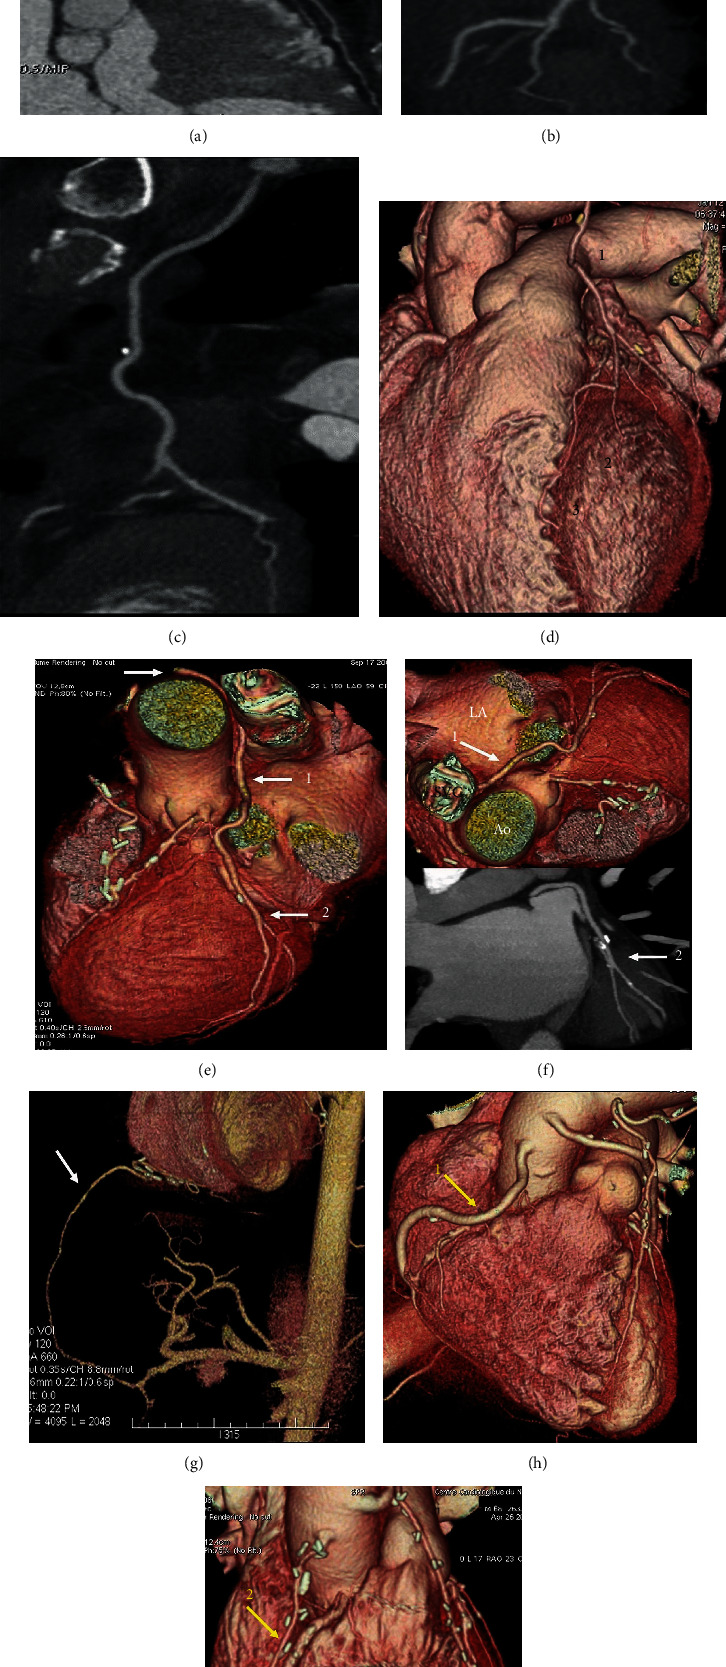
(a–i) Postprocessing of CT angiography of CABG by mean of volume rendering and 2D curved imaging with automatic tracking. (a–c) show 2D curved imaging of CABG with automatic tracking. (a) LITA anastomosed on LAD. (b) LITA sequential grafting on LAD and first diagonal branch. (c) RITA anastomosed on first obtuse branch. (d-i) Volume rendering imaging of CABG. (d) 1-LITA grafted on 2-Diag branch and 3-LAD. (e, f) RITA grafted on CCA. White arrow (1) RITA runs between the aorta and LA, (2) distal grafting on first obtuse branch. (g–i) Comparison between second target conduit on RCA. (g) White arrow gastroepiploic anastomosed to the PDA. (h, i) Yellow arrow (1) SVG and (2) RAG. Note that the venous graft size is greater than the arterial graft size. CABG: coronary artery bypass grafting; LITA: left internal thoracic artery; LAD: left anterior descending; RITA: right internal artery; CCA: circonflexe coronary artery; LA: left appendix; RCA: right coronary artery; PDA: posterior descending artery; SVG: saphen vein graft; RAG: radial artery graft.
